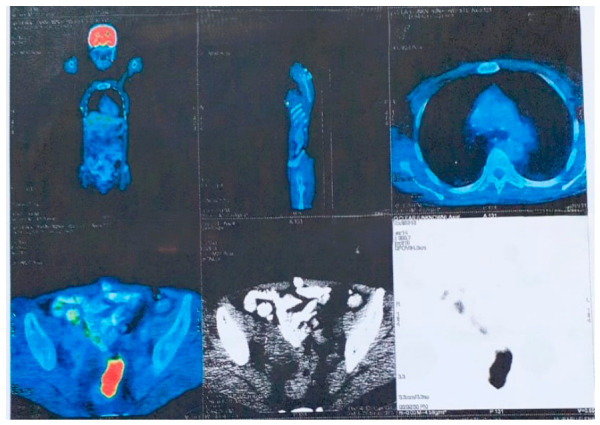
Patient nr 66—Pet CT showing metabolic activity in the rectum.
